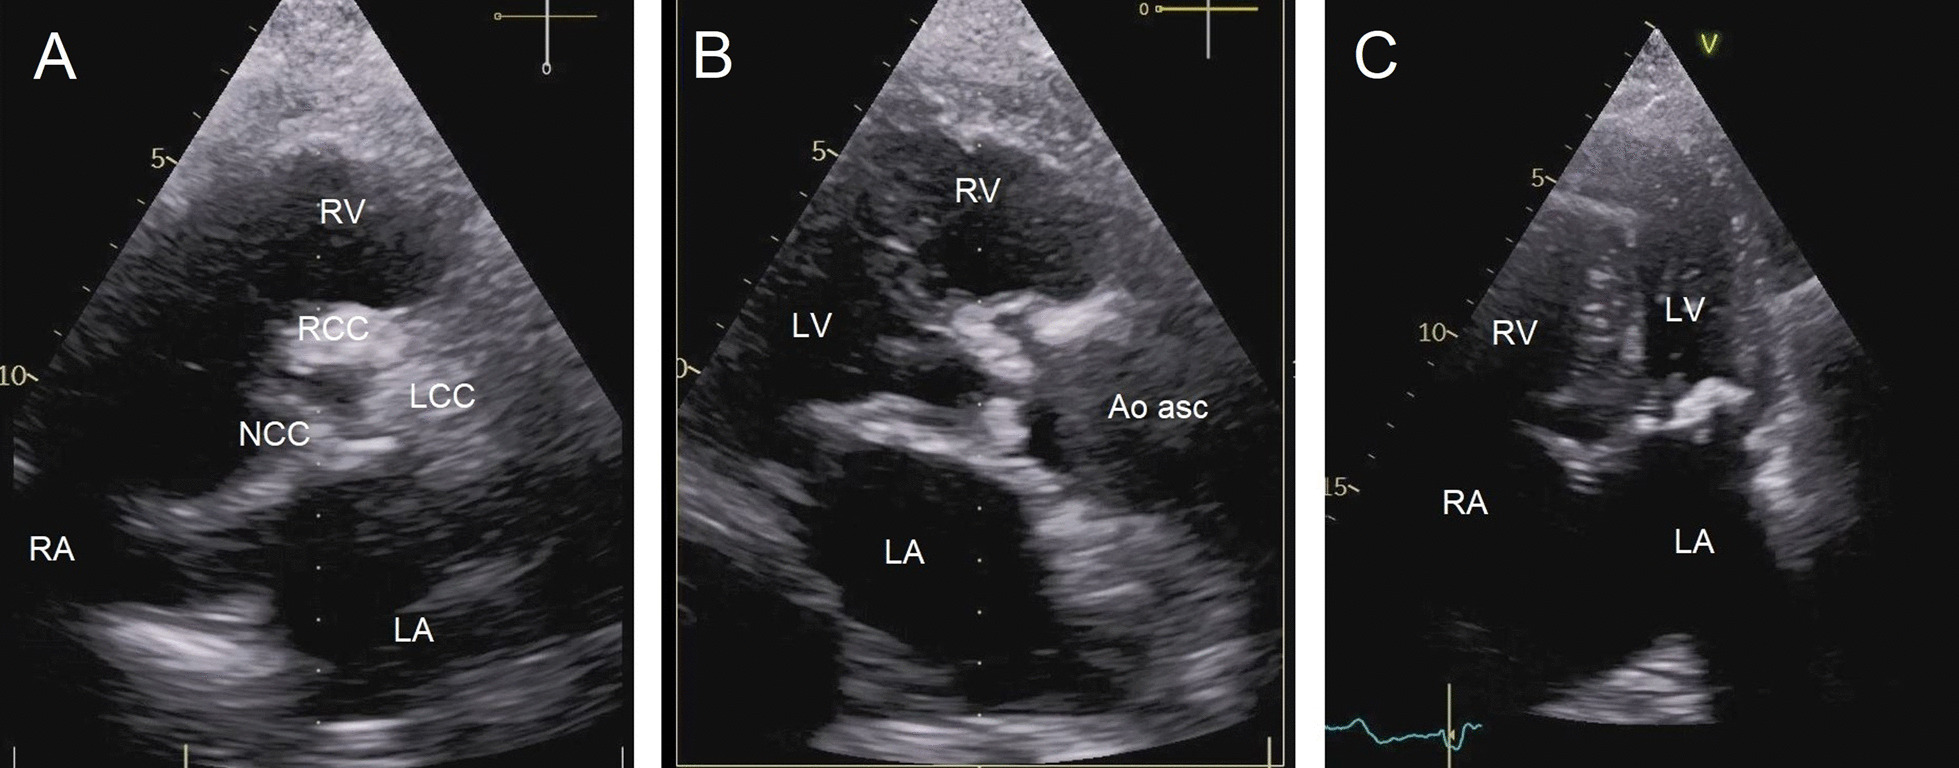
Preoperative transthoracic echocardiogram images. Parasternal short-axis view (A) and parasternal long-axis view (B) of the calcified aortic valve. Apical four-chamber view showing left ventricular hypertrophy and severe calcification of the mitral valve (C). Ao asc (ascending aorta), LA (left atrium), LCC (left coronary cusp), LV (left ventricle), NCC (non-coronary cusp), RA (right atrium), RCC (right coronary cusp), RV (right ventricle)
